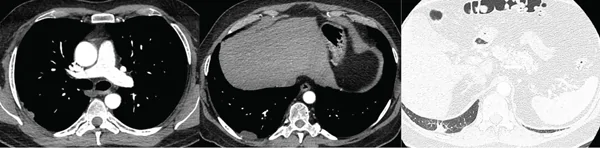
(a) Preoperative chest computed tomography
